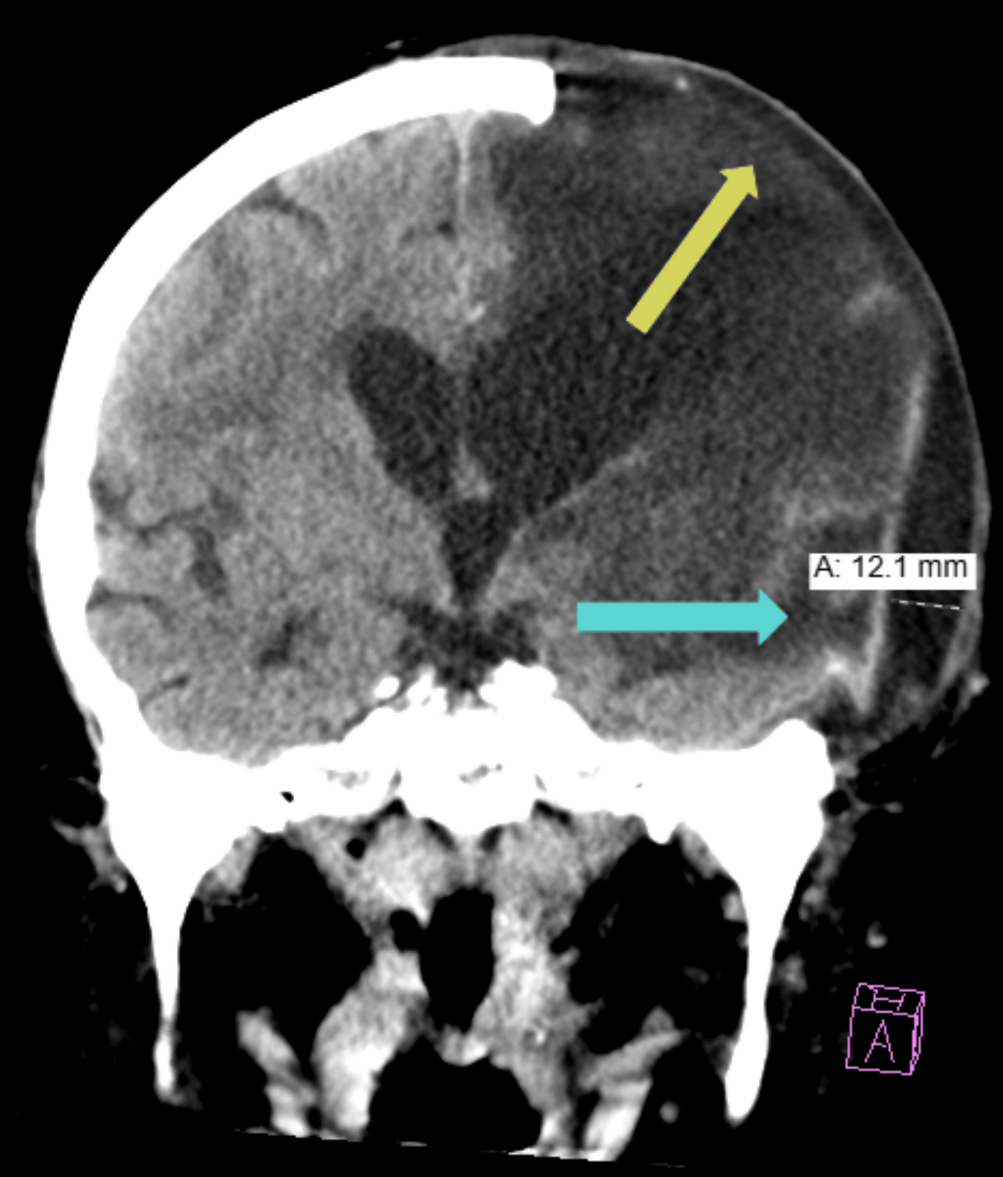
Noncontrast CT Head Redemonstrated postoperative changes of decompressive left hemicraniectomy with persistent mass effect and transcalvarial brain herniation (yellow arrow), similar to the prior examination. There is an interval increase in the size of the hypodense extra-axial fluid collection (blue arrow) subjacent to the craniectomy site, measuring up to 12 mm in greatest thickness, previously measured at 5 mm on MRI brain dated September 17, 2024. No significant midline shift.
